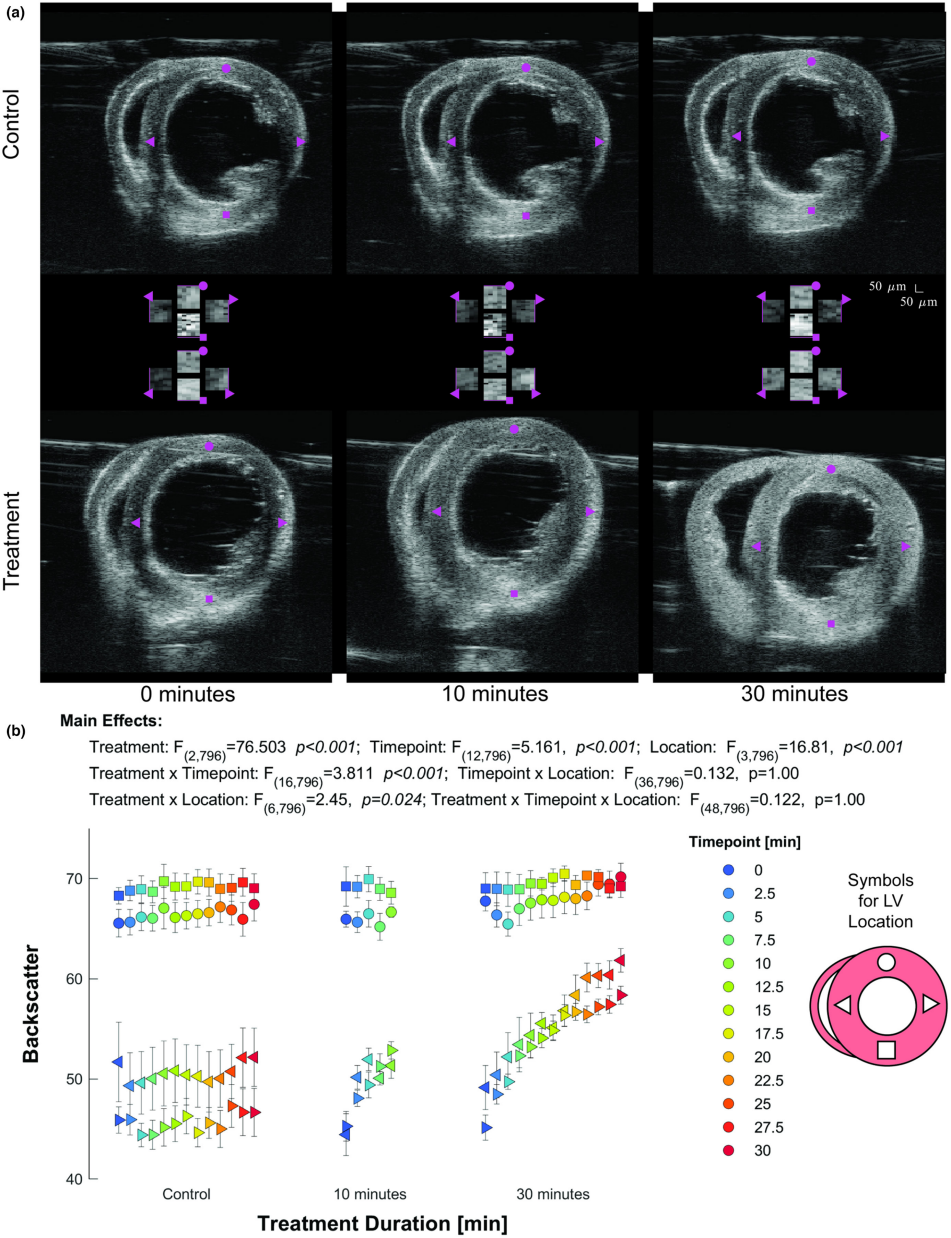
Effect of Collagenase Treatment on Ultrasonic Backscatter. (a) Mid‐myocardial short‐axis ultrasonic backscatter of one control (top) and one collagenase treated (bottom) heart at three time points. Horizontal center represents the generalized backscatter at each of the four cardinal positions analyzed. The LV positions as shown on the inset for (b) are: ●‐anterior myocardium; ►‐lateral myocardium; ■‐ posterior myocardium, ◄‐septal myocardium. Scale bar shows 50 μm square for full short‐axis views (top/bottom); center images are each 25 μm square. (b) Statistical analysis. Treatment duration and myocardial position show a significant interaction, with 30 min data suggesting an increase in backscatter intensity in myocardial sections that align parallel to the angle of insonification, and indicate minimal alteration in backscatter intensity in myocardial locations that are perpendicular to the direction of insonification. Treatment and treatment duration show a significant interaction, with controls showing little or no change in intensity over 30 min, while collagenase treatment increases backscatter intensity of septal and lateral wall positions (triangles) for each timepoint.
